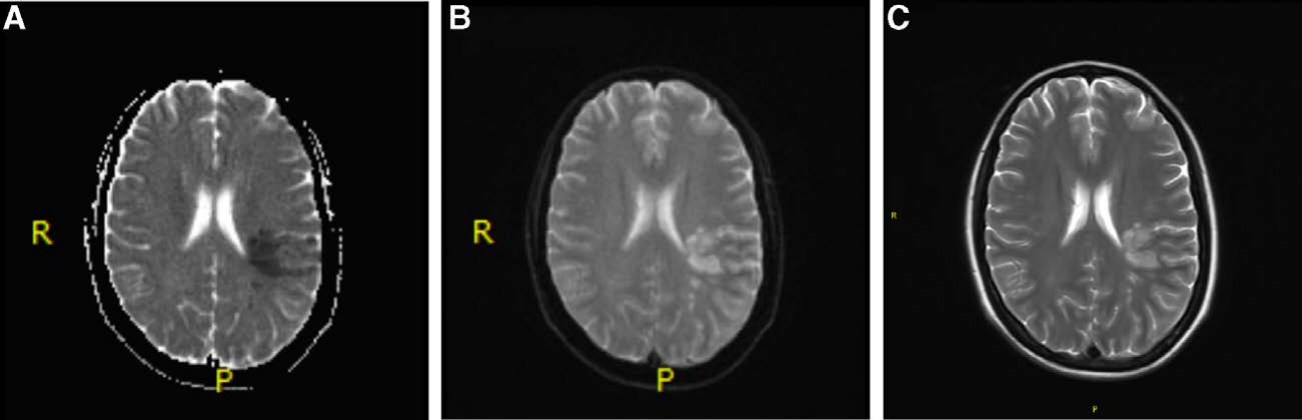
Brain magnetic resonance imaging showed cerebral infarction in the left parietal temporal lobe (A: Apparent Diffusion Coefficient sequence, B: Diffusion weighted imaging, C: T2 weighted imaging).
